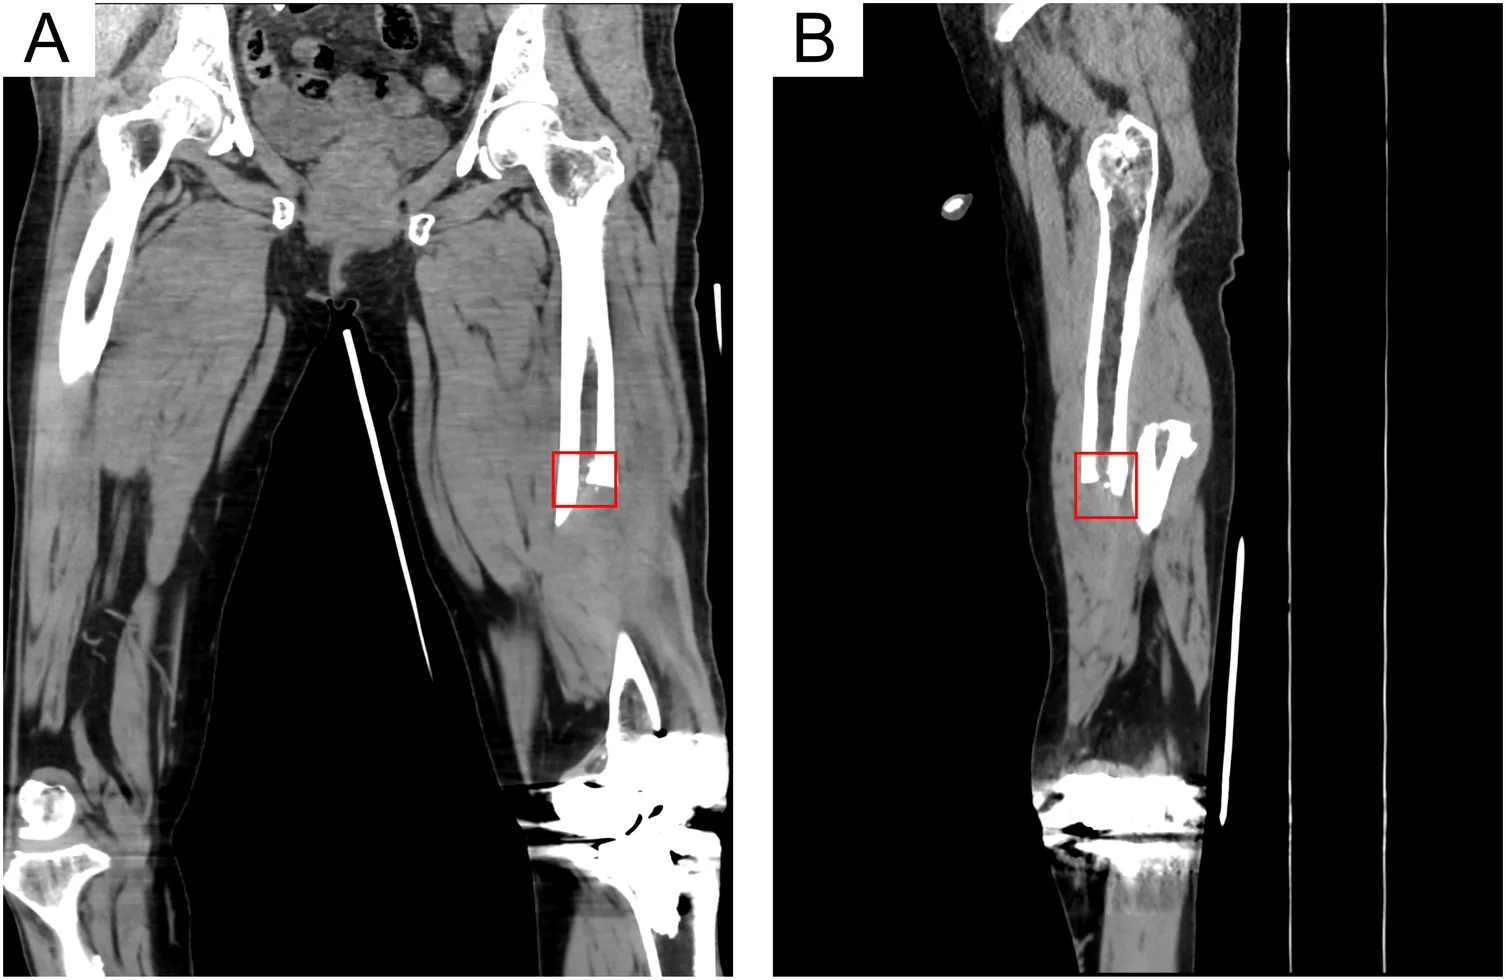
Preoperative CT imaging retrospectively identifying the bone cement plug. (A,B) Coronal and sagittal CT images, respectively, with red boxes highlighting the suspected cement plug within the medullary canal, which was not appreciated on initial interpretation but was later confirmed intraoperatively.
